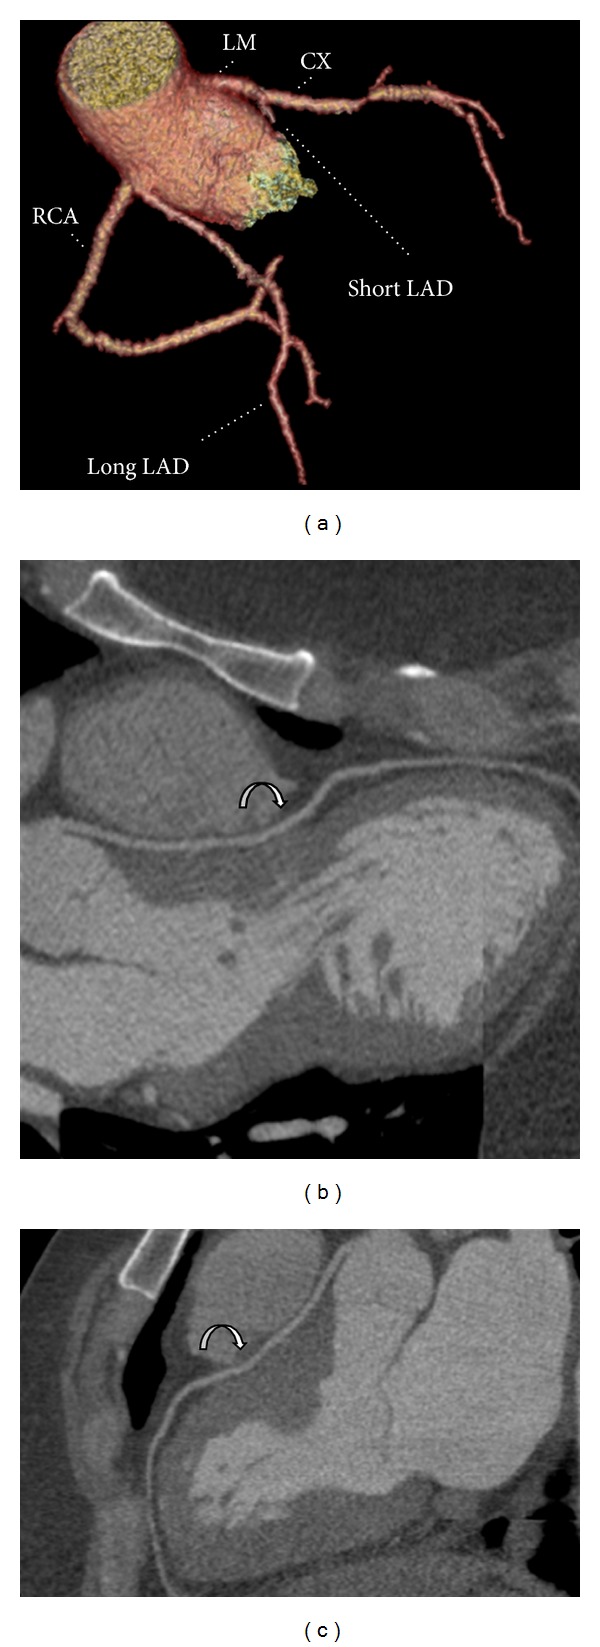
(a) 3D volume-rendered CTA images of the coronary circulation (LM: left main coronary artery; CX: circumflex branch; LAD: left anterior descending artery; RCA: right coronary artery). (b) and (c): Curved multiplanar reconstruction CTA images of the long LAD arising from the right coronary cusp with hammock-like descending curvature (curved arrows) consistent with a transseptal course.
